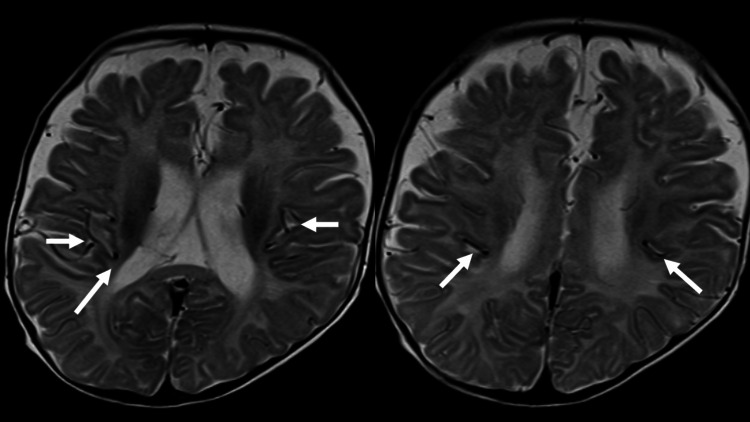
Case 1: T2-weighted images. The middle cerebral artery branches are tortuous in the sulci near the central sulcus (arrows).
